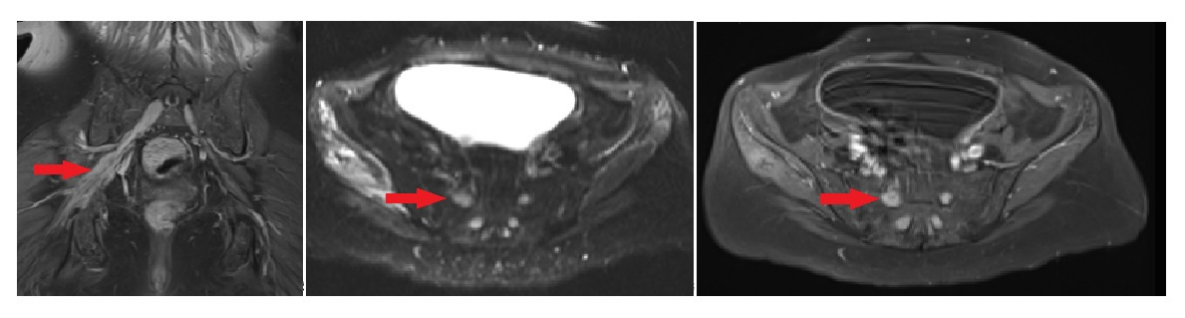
MRI of pelvis T1-weighted coronal T1 images and axial post-contrast images demonstrate S1 and sacral plexus enlargement (see arrow), and T2-weighted axial images demonstrate hyperintense signal within the right gluteus minimus and medius musculature.
